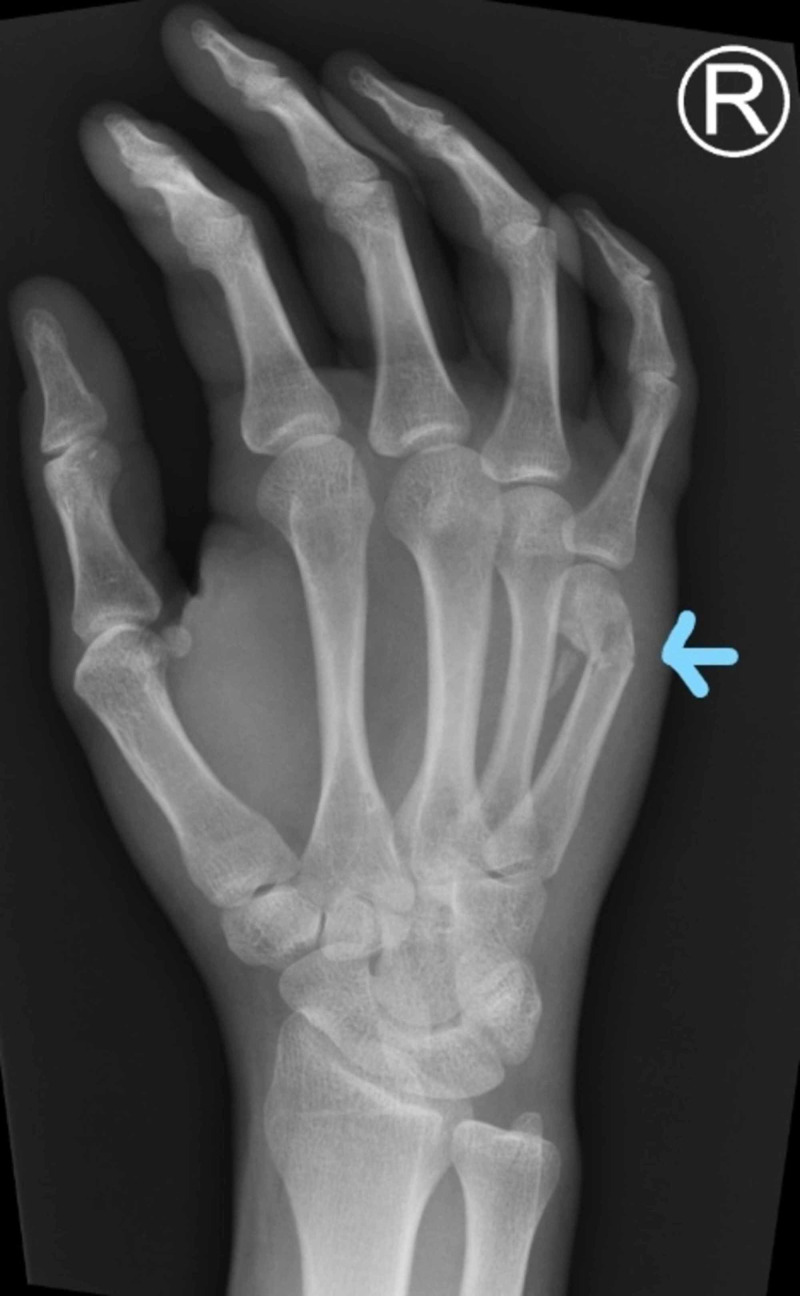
X-ray (oblique view) shows an extra-articular, comminuted fracture of the neck of the fifth metacarpal with volar angulation
